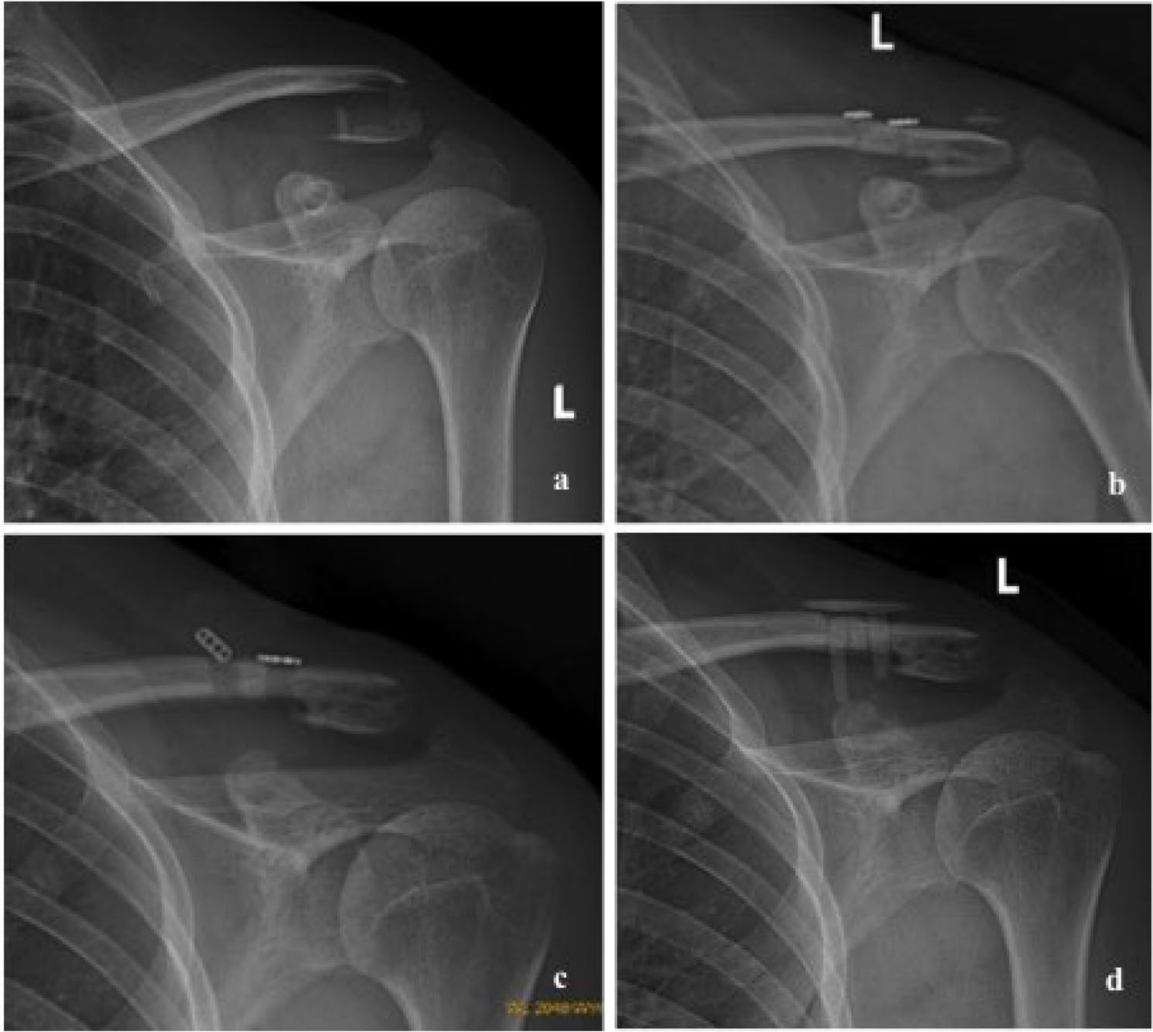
a A 31-year-old men with left distal clavicle fracture. b X-ray film showed a good reduction of clavicle fracture after surgery. c X-ray film showed fixation failure of medial button plate after 2month postoperatively. d It had healed completely by use of hardware removal and antibiotic spacer implantation at 3 months postoperatively
